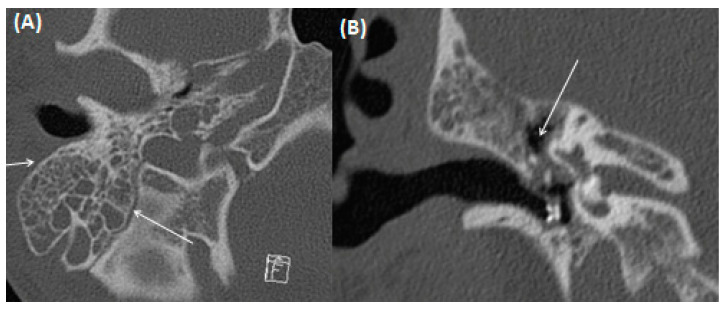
CT of a 17-year-old male patient using bone windowing with axial (A) and coronal (B) views of the right middle ear. Complete obstruction of the mastoid cells is present without multiple bony sclerosis. A tympanic tube through the thickened eardrum has been placed. Obstruction of the epitympanum without bony erosion of the scutum is present.
